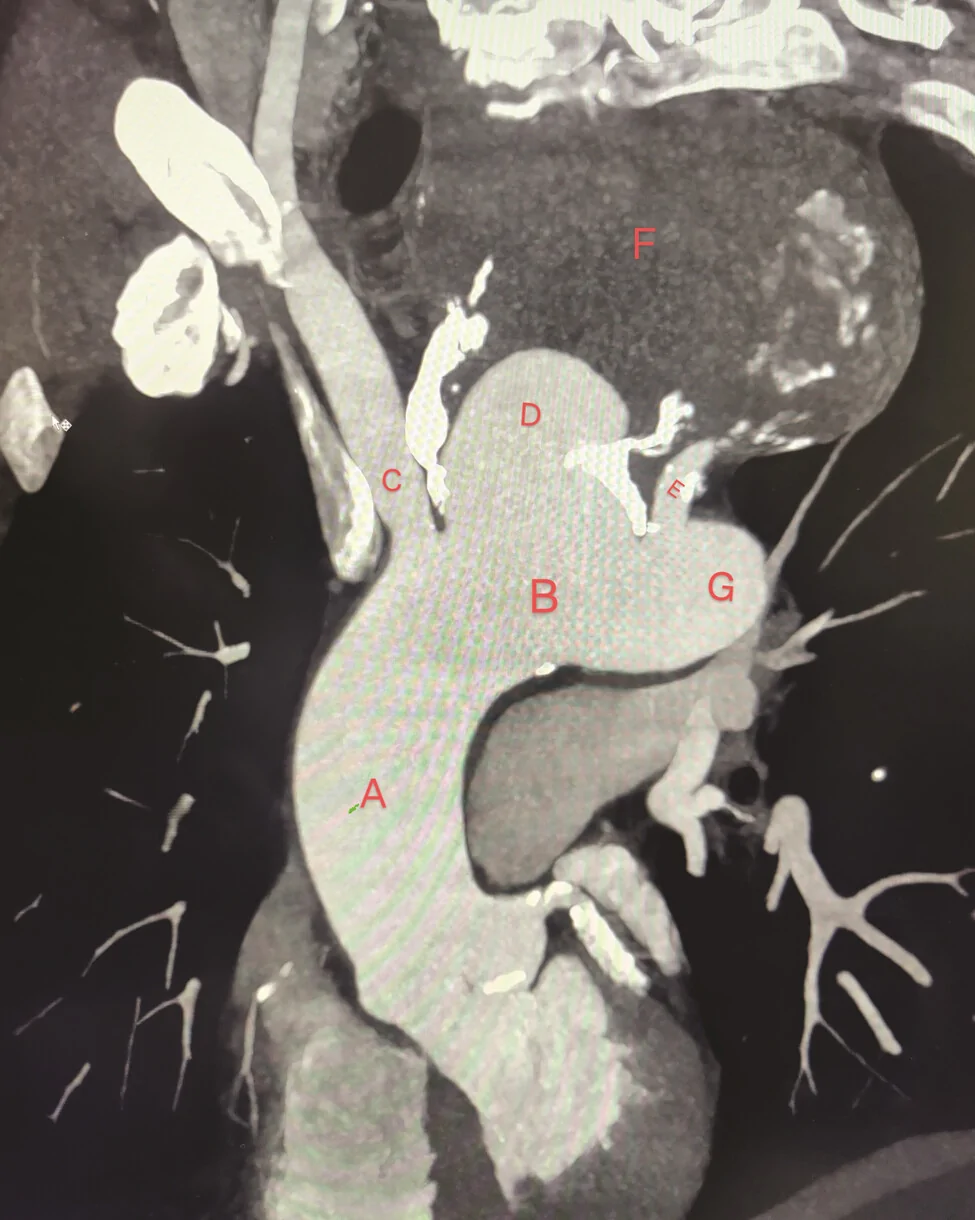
CT aortogram—aortic arch pseudoaneurysm with peripheral calcification (porcelain arch) and partial thrombosis. Labels: A, ascending aorta; B, aortic arch; C, innominate artery; D, pseudoaneurysm; E, left subclavian artery; F, thrombosed lumen; G, distal arch. CT, computed tomography.
